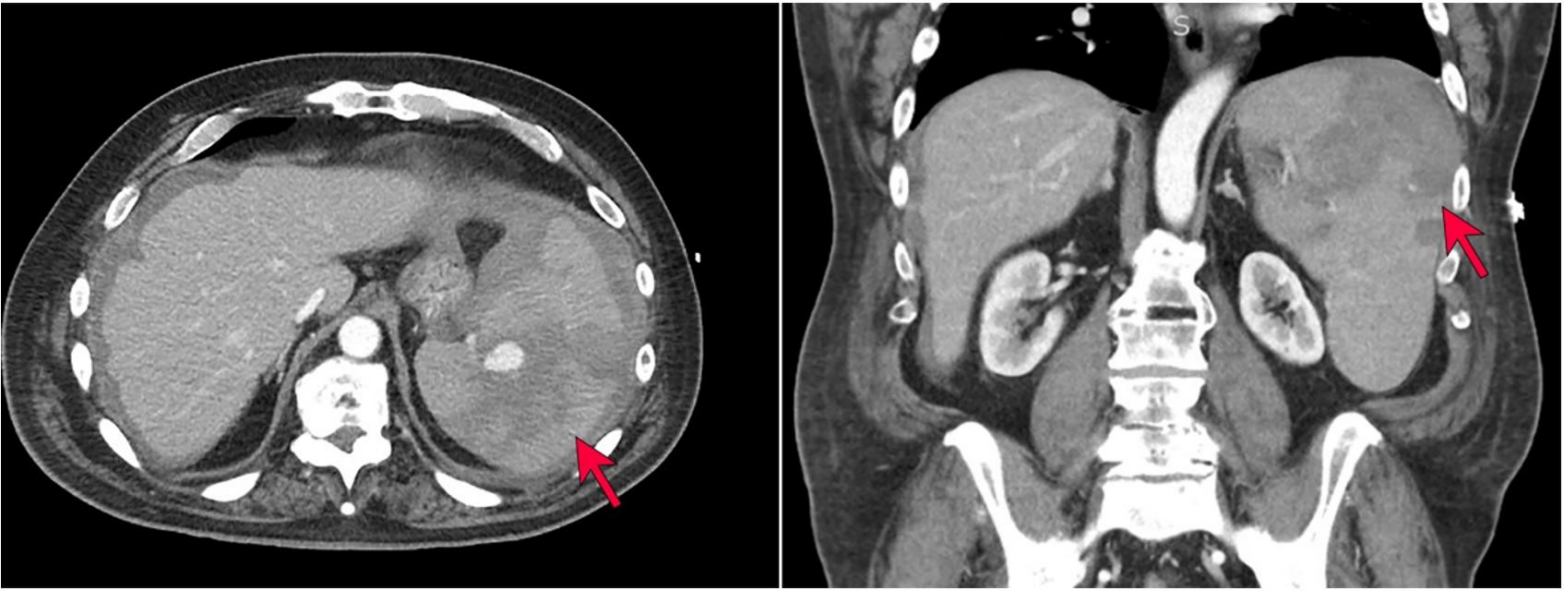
CT imaging demonstrating splenomegaly with hemorrhage, splenic laceration, and perisplenic hematoma (red arrows). Lymphadenopathy was also noted on imaging (not shown).
